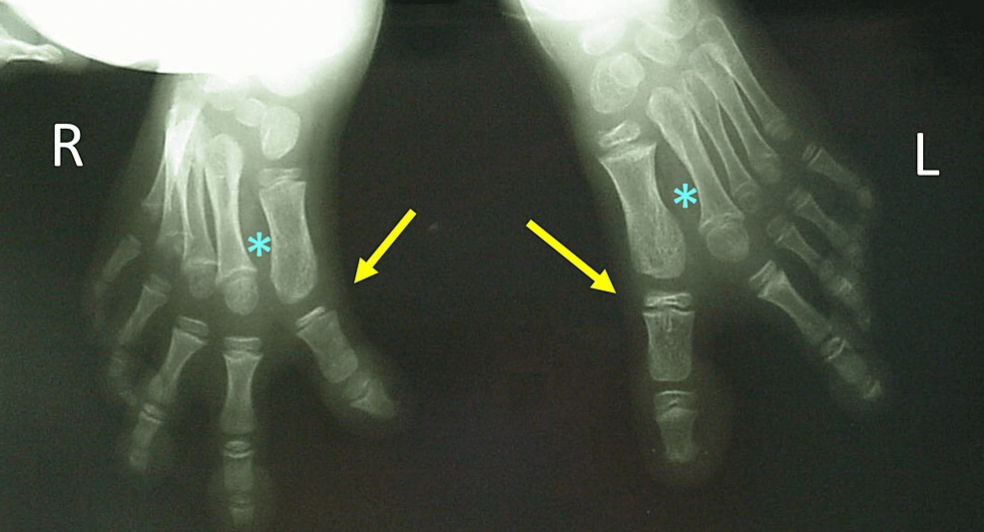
Anteroposterior x-ray view in a 3-year-old girl with bilateral congenital hallux varus The level of the deformity is at the first metatarsophalangeal joint (yellow arrows). The intermetatarsal angle is normal (asterisk)
